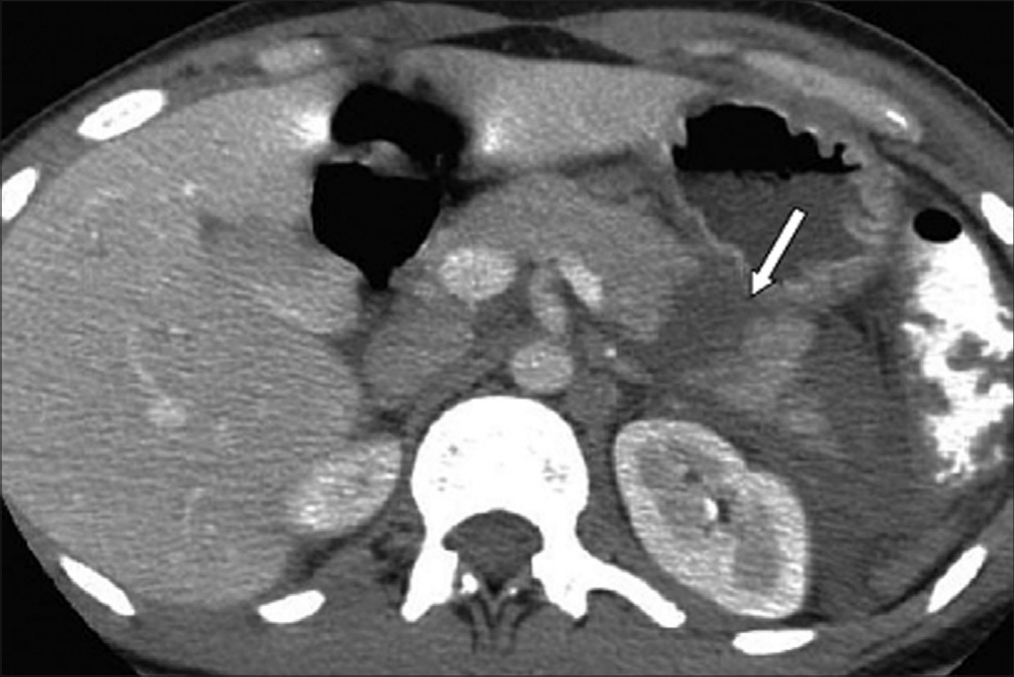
A computed tomography scan revealing a transection through the tail of the pancreas
